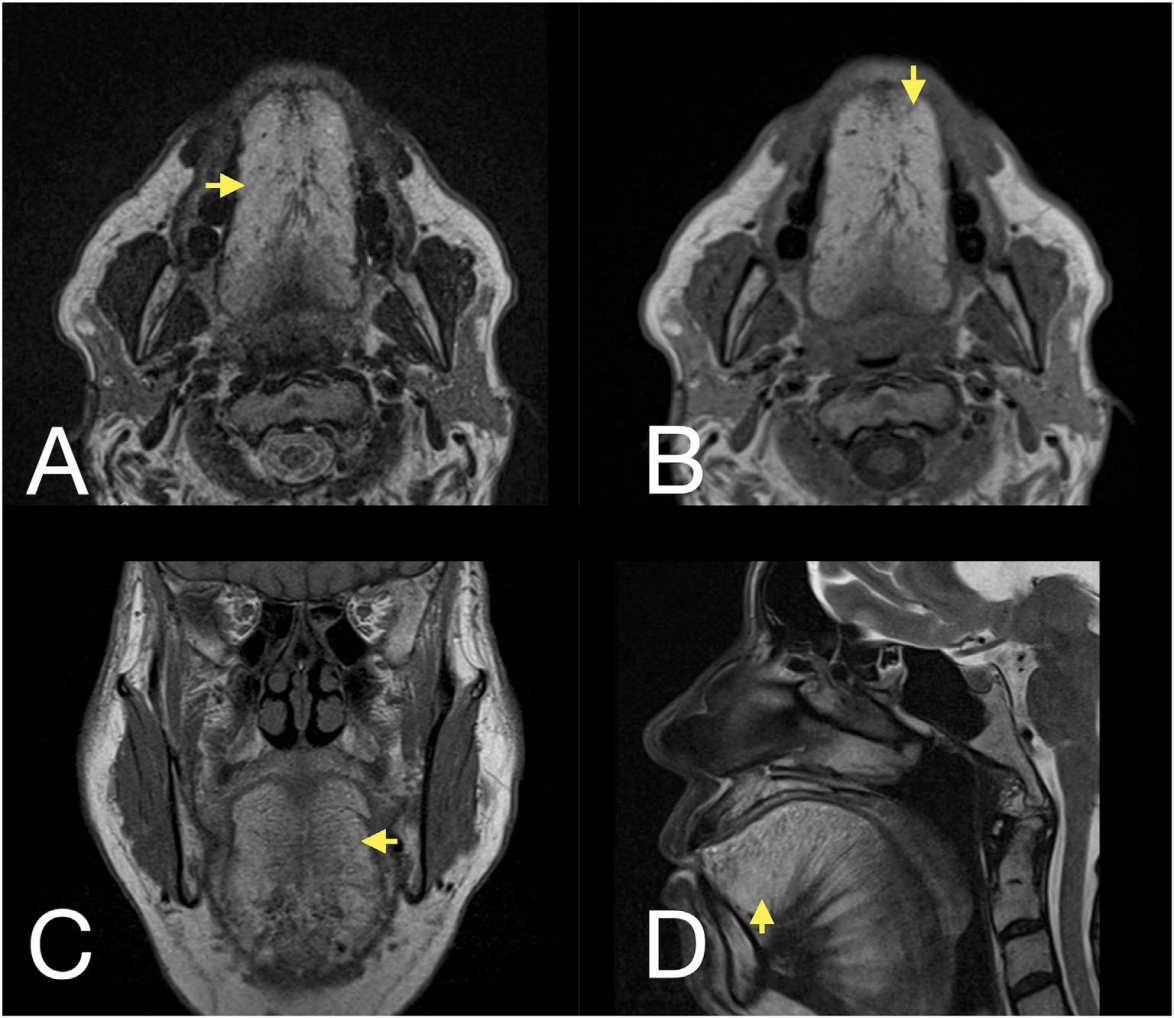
(A) Axial T2‐weighted MR image showing the tongue with a marked reduction in volume following symmetrical bilateral glossectomy, with persistent high signal intensity (arrowheads) typical of adipose tissue. (B) Axial T1‐weighted MR image showing a similar high signal intensity pattern. (C) Coronal T1‐weighted MR image confirming persistent high signal intensity (arrowheads). (D) Sagittal T2‐weighted MR image demonstrating residual benign symmetrical lipomatosis (BSL) with high signal intensity (arrowheads) in a tongue reduced by approximately 50% postoperatively.
